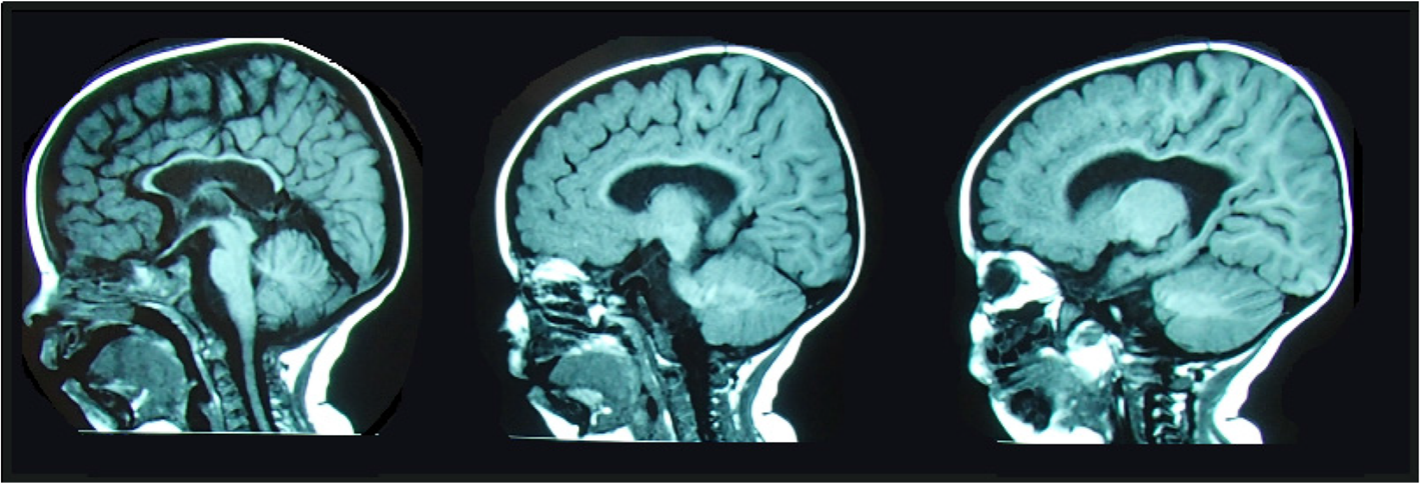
Magnetic resonance imaging of the brain evidencing corpus callosum hypoplasia
